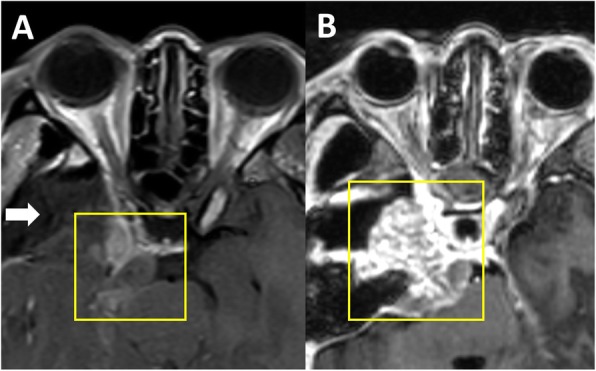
Radiographic Images of Sinonasal Carcinoma following intracranial surgical resection. Magnetic resonance images in the axial planes are presented. There was a gross total resection of the brain metastasis (as denoted by the white arrow) but progression of tumor in the prepontine cistern ventral to the brain stem (a). Two weeks later, planning imaging demonstrates further progression (b)
